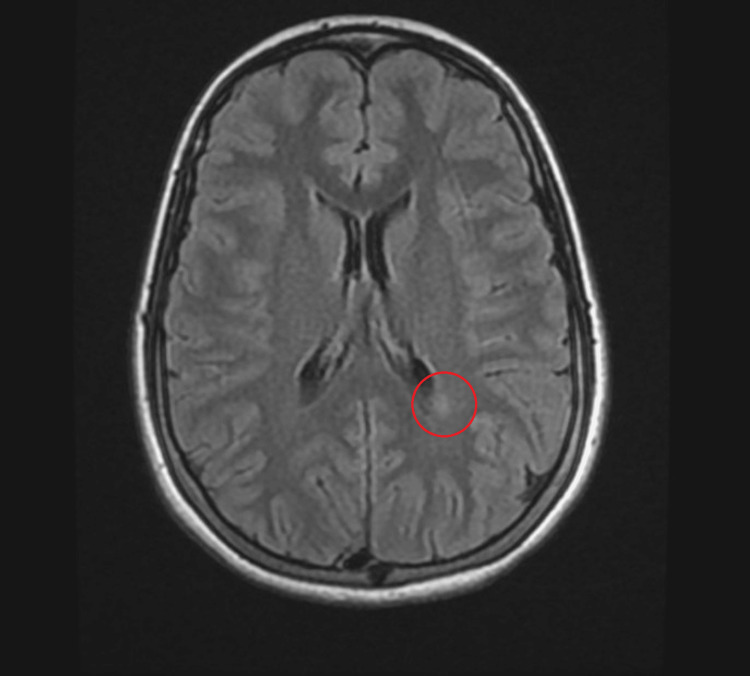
Cranial MRI findings (axial T2-FLAIR sequences) demonstrating a subtle posterior periventricular hyperintense lesion (indicated by the red circle). While the brainstem appeared radiographically normal, this periventricular finding in an adolescent patient serves as a critical indicator of a potential early demyelinating process, supporting the clinical suspicion of multiple sclerosis or clinically isolated syndrome. FLAIR: Fluid-Attenuated Inversion Recovery
